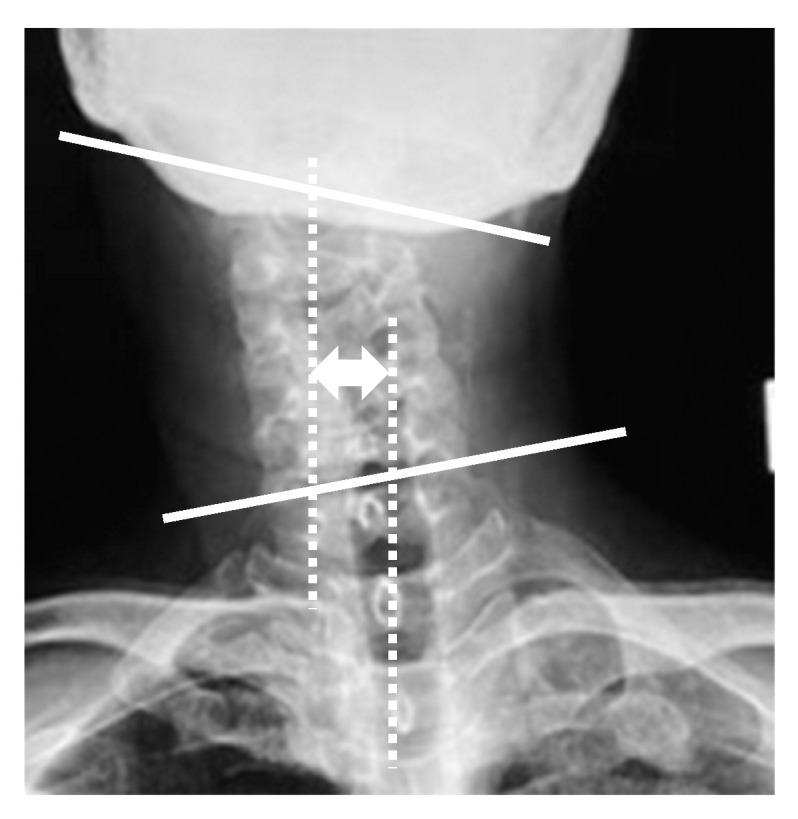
Radiographic parameters: cervicomandibular angle and C2 to T1 distance.
